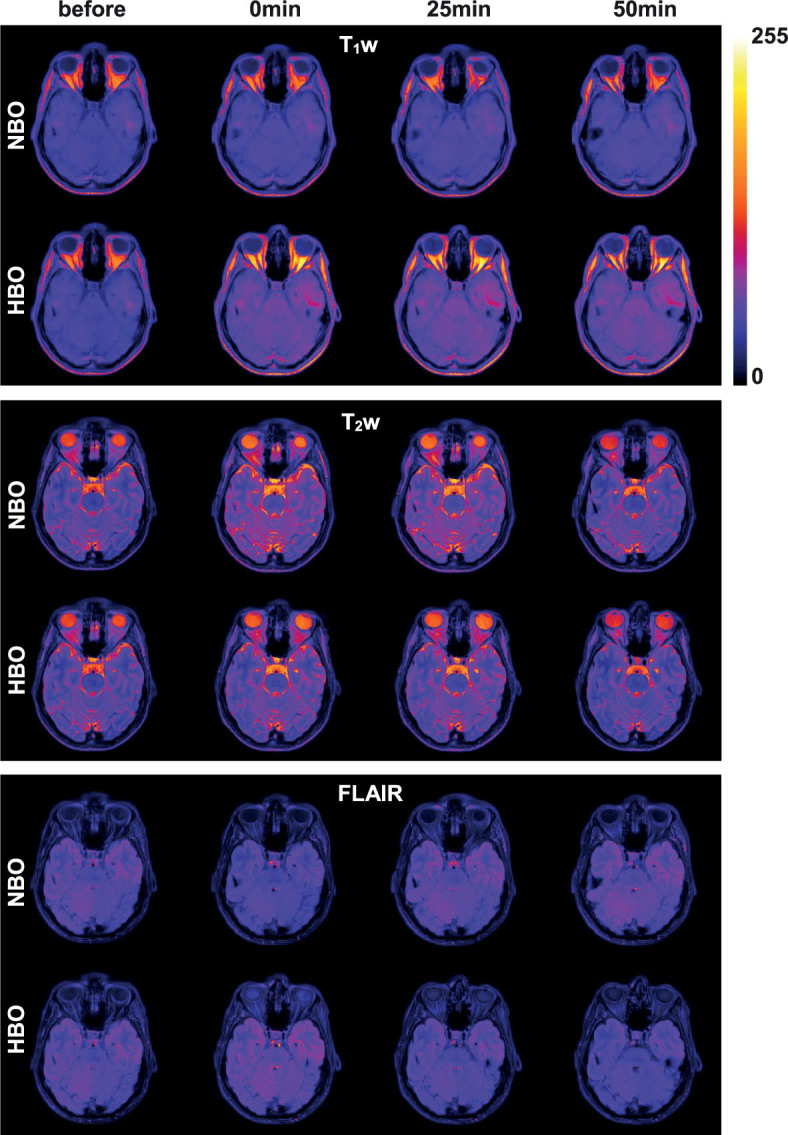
Representative MRI images in healthy subject at baseline, immediately after the end, after 25 min and after 50 min of NBO or HBO.
